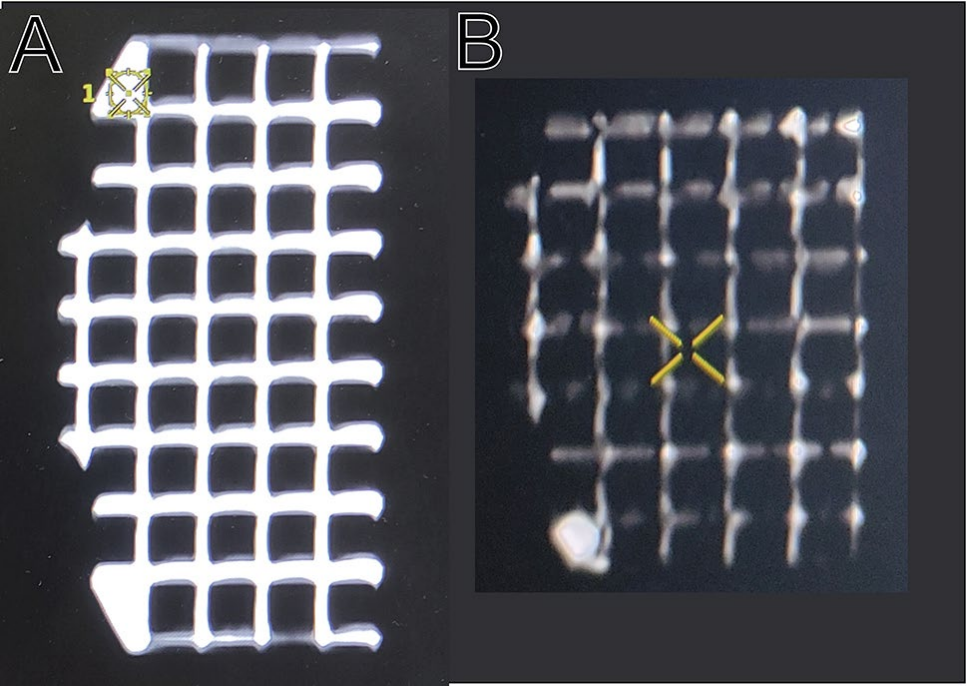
Differences in visibility in MRI scans between a grid insert prototype fully filled with liquid (A) and one which is only partially filled with liquid (B)
